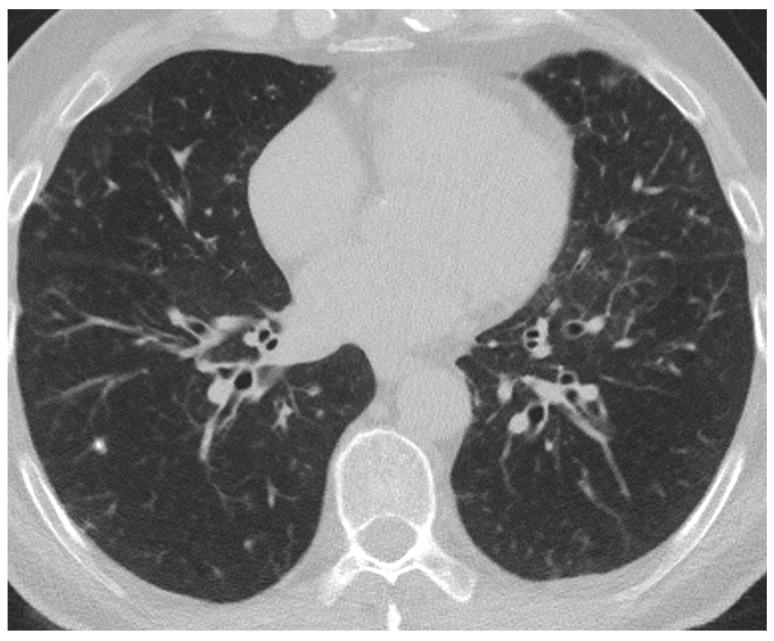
Chest CT: diffuse nodular infiltrates in the right lung, with areas of calcification in the superior segment of the right lower lobe, consistent with pulmonary histoplasmosis. Representative image from the anonymized institutional radiology archive of University Hospital Frankfurt.
